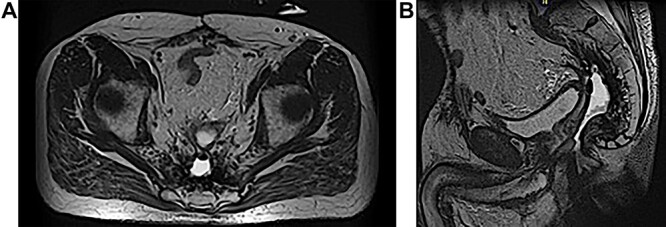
(A and B) MRI abdomen and pelvis demonstrating 2–3 cm pelvic sinus cavity with connecting tract to the perineum.
